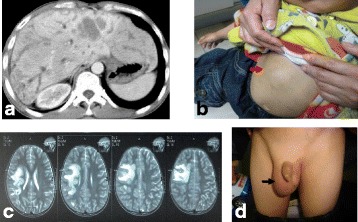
Damage caused by the main two parasitic infections. a CT image of intrahepatic biliary dilatations caused by *Clonorchis sinensis* infection. b MRI of brain cyst due to cerebral paragonimiasis. c Patient presented with a subcutaneous nodule in his right upper abdomen (*red arrow*) due to cutaneous paragonimiasis. d A patient presented with a mass in his right scrotum (*black arrow*) due to cutaneous paragonimiasis. All pictures are reproduced with the permission of the patient or that of his or her guardians
